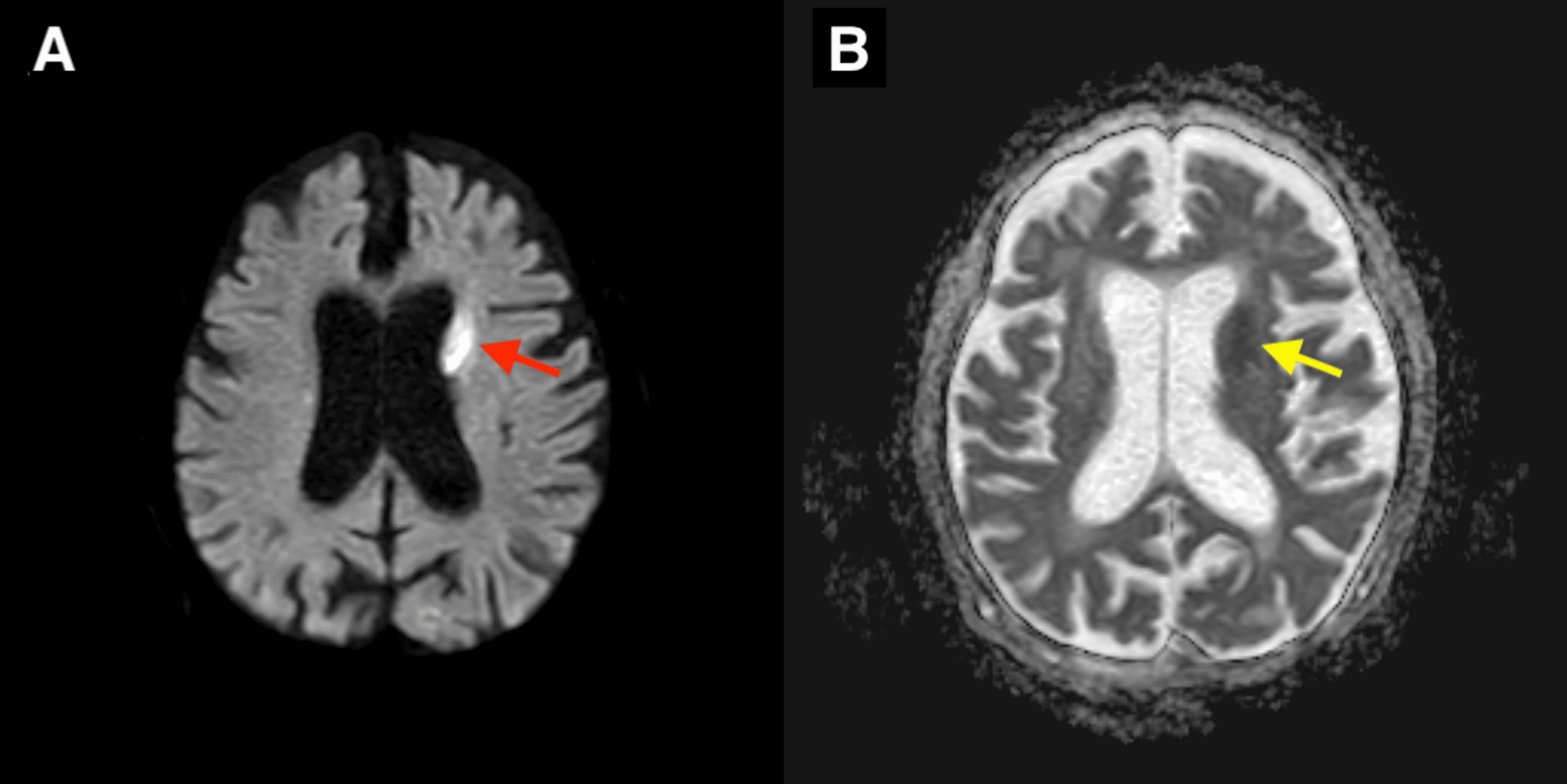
Brain magnetic resonance imaging (MRI) A brain MRI was performed on hospital day 6. Axial diffusion-weighted image (DWI) (A) reveals a hyperintense acute infarct in the left caudate nucleus (red arrow), and the corresponding apparent diffusion coefficient (ADC) map (B) demonstrates a hypointense signal in the same region (yellow arrow).
